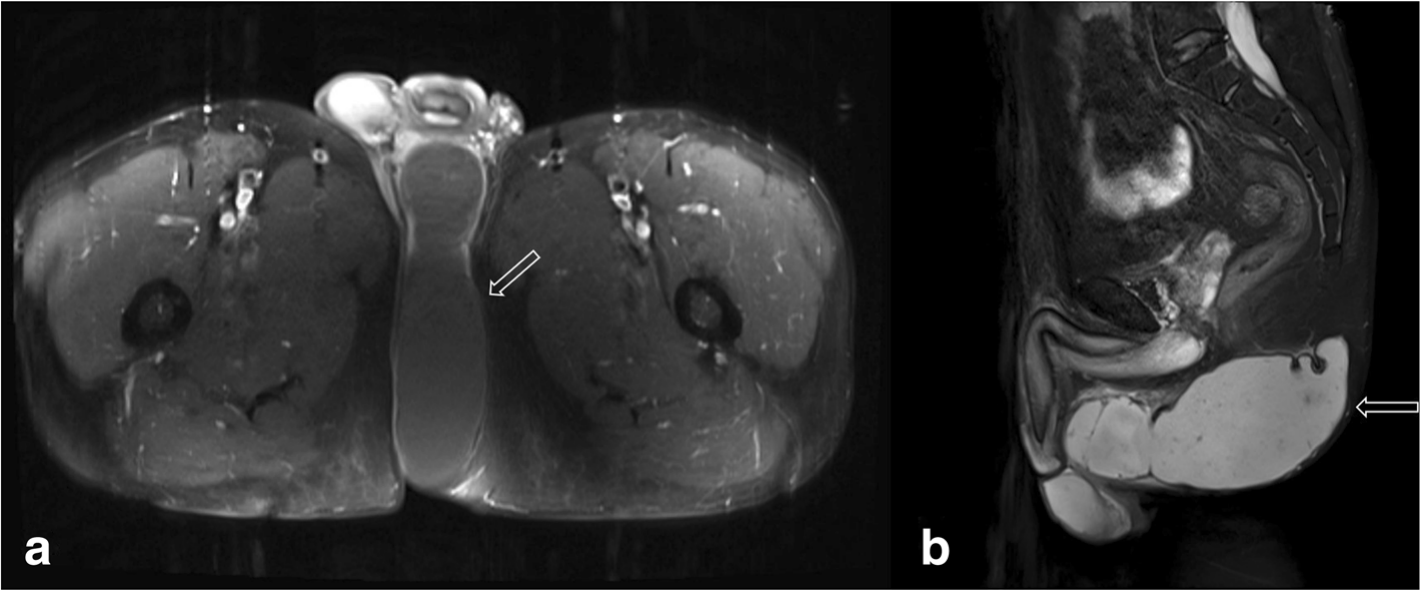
Magnetic resonance imaging findings. The image shows a 6.7 × 16 cm lobulated mass in the medial aspect of the left perineum. a Transverse view. b Sagittal view
